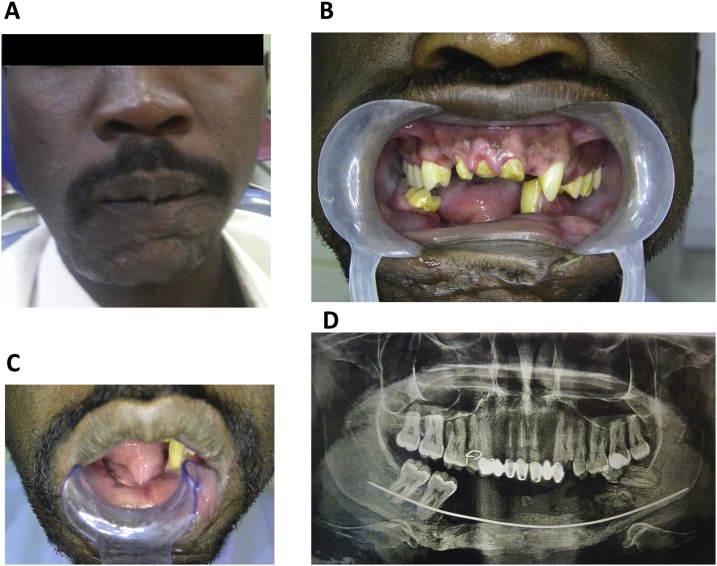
Patient’s clinical presentation. A. The patient’s photograph presents his frontal view at the time of presentation with a slight facial deformity and multiple scars on the submental region. B. The intraoral view presenting severely destroyed crowns in teeth 12, 11, and 21; missing teeth 13, 22, 45, 44, 43, 42, 41, 31, and 32; and a fractured crown in tooth 38. C. The intraoral view of the patient with an obliterated sulcus. D. The panoramic radiograph shows the  presence of a supporting wire and a fracture in crown 38.
